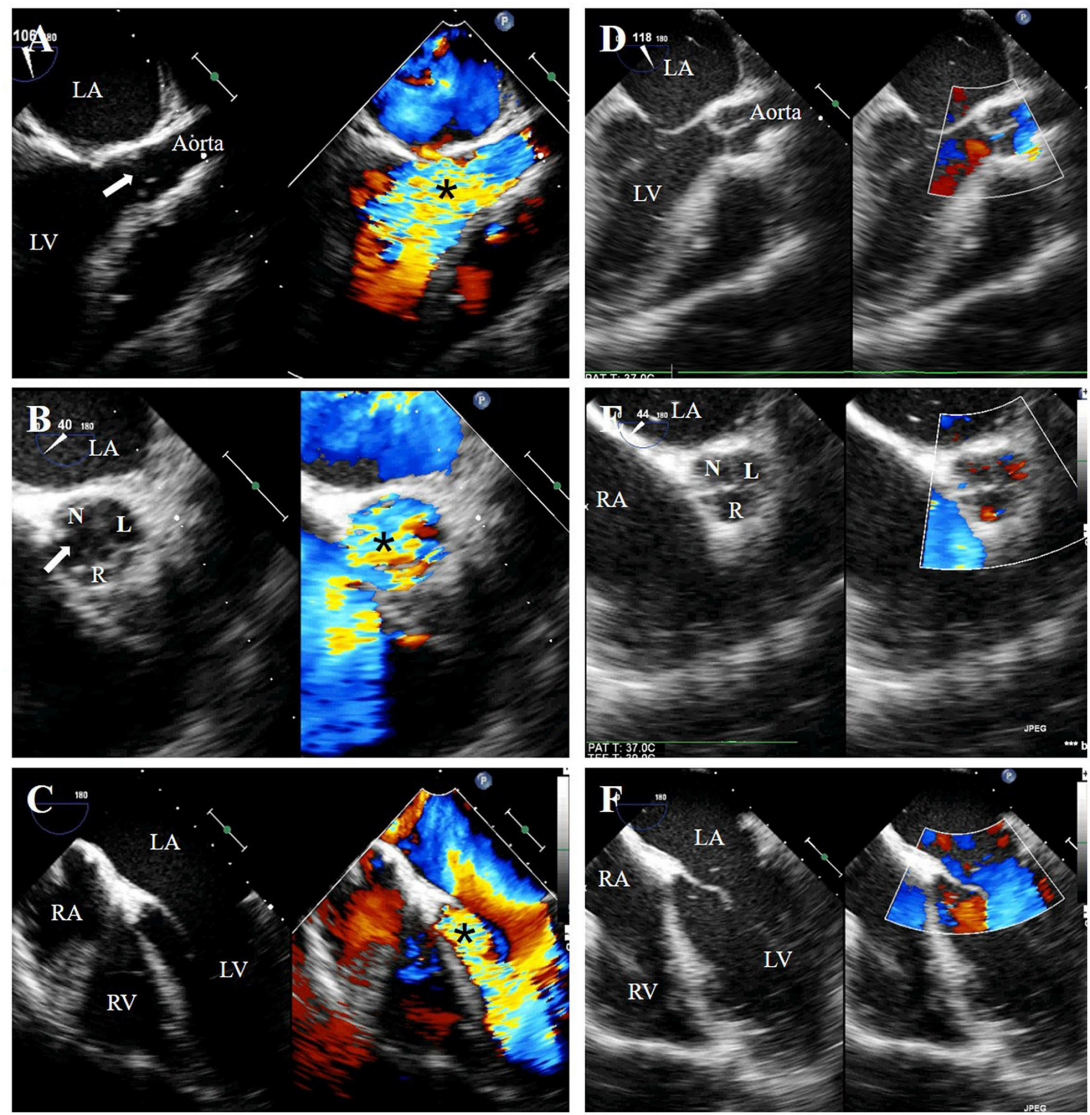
Preoperative transesophageal echocardiography (TEE) showed free-flow aortic regurgitation (black asterisk), which is caused by a fissure (white arrow) formed between the flailed right coronary cusp and non-coronary cusp, on the long-axis view (A), short-axis view (B) and five-chamber view (C). Postoperative TEE showed well-functioning new valves without regurgitation (D–F). N, non-coronary cusp; R, right coronary cusp; L, left coronary cusp; LA, left atrium; RA, right atrium; LV, left ventricle; RV, right ventricle.
